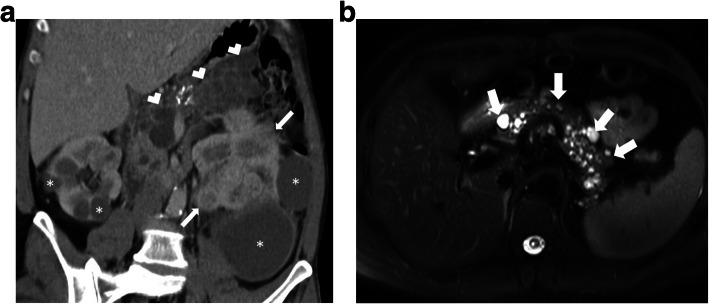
Two different patients with VHL. a Coronal plane post-contrast CT image of a 47-year-old male patient shows innumerable pancreatic cysts (arrowheads). Also note is made of large-sized solid RCC (arrows) in the left kidney and bilateral renal cysts (asterisks). b Axial plane T2W MR image of a 40-year-old male patient demonstrates multiple cysts of the pancreas, almost completely replacing pancreatic parenchyma (arrows)
